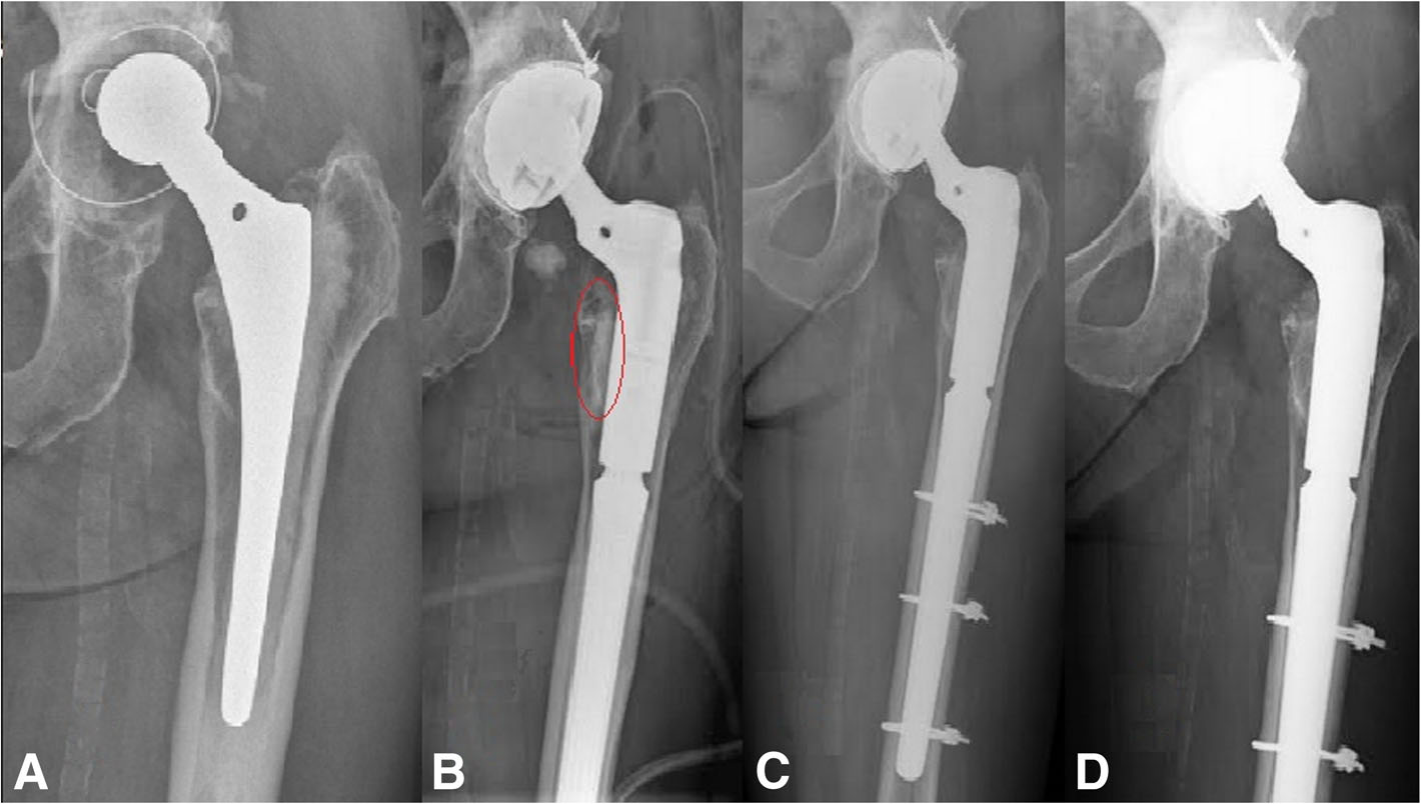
Revision of THA using a cementless femoral component. Preoperative image shows unstable components (a). The proximal part of the femur postoperatively (b) shows CERAMENT™|G present (circled in red). In the X-ray after 3 months (c), the bone graft substitute is difficult to differentiate from bone. Image after 1 year (d) shows bone healing
